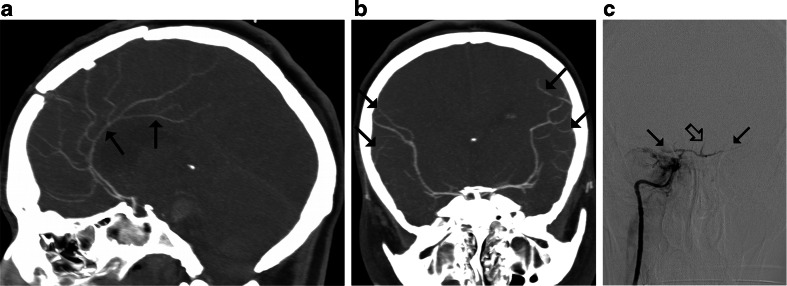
CTA findings in a 34-year-old woman (patient no. 44) with brain stem hematoma and frontal craniotomy presenting signs of BD on clinical examination. a Ten millimeter MIP in sagittal plane. CTA shows opacification of both pericallosal arteries (*thin arrows*); b Ten millimeter MIP in coronal plane. CTA shows opacification of the cortical segments of the MCAs (*thin arrows*); these findings exclude the diagnosis of BD according to the 10-, 7-, and 4-point scales. c Catheter angiography of the right ICA performed 3 h later revealed delayed, residual filling of the M1 segments of the MCAs (*thin arrows*) and the A2 segments of the ACAs (*thick arrow*) that occurred 18 s after injection. This result was interpreted as stasis filling consistent with the diagnosis of BD
